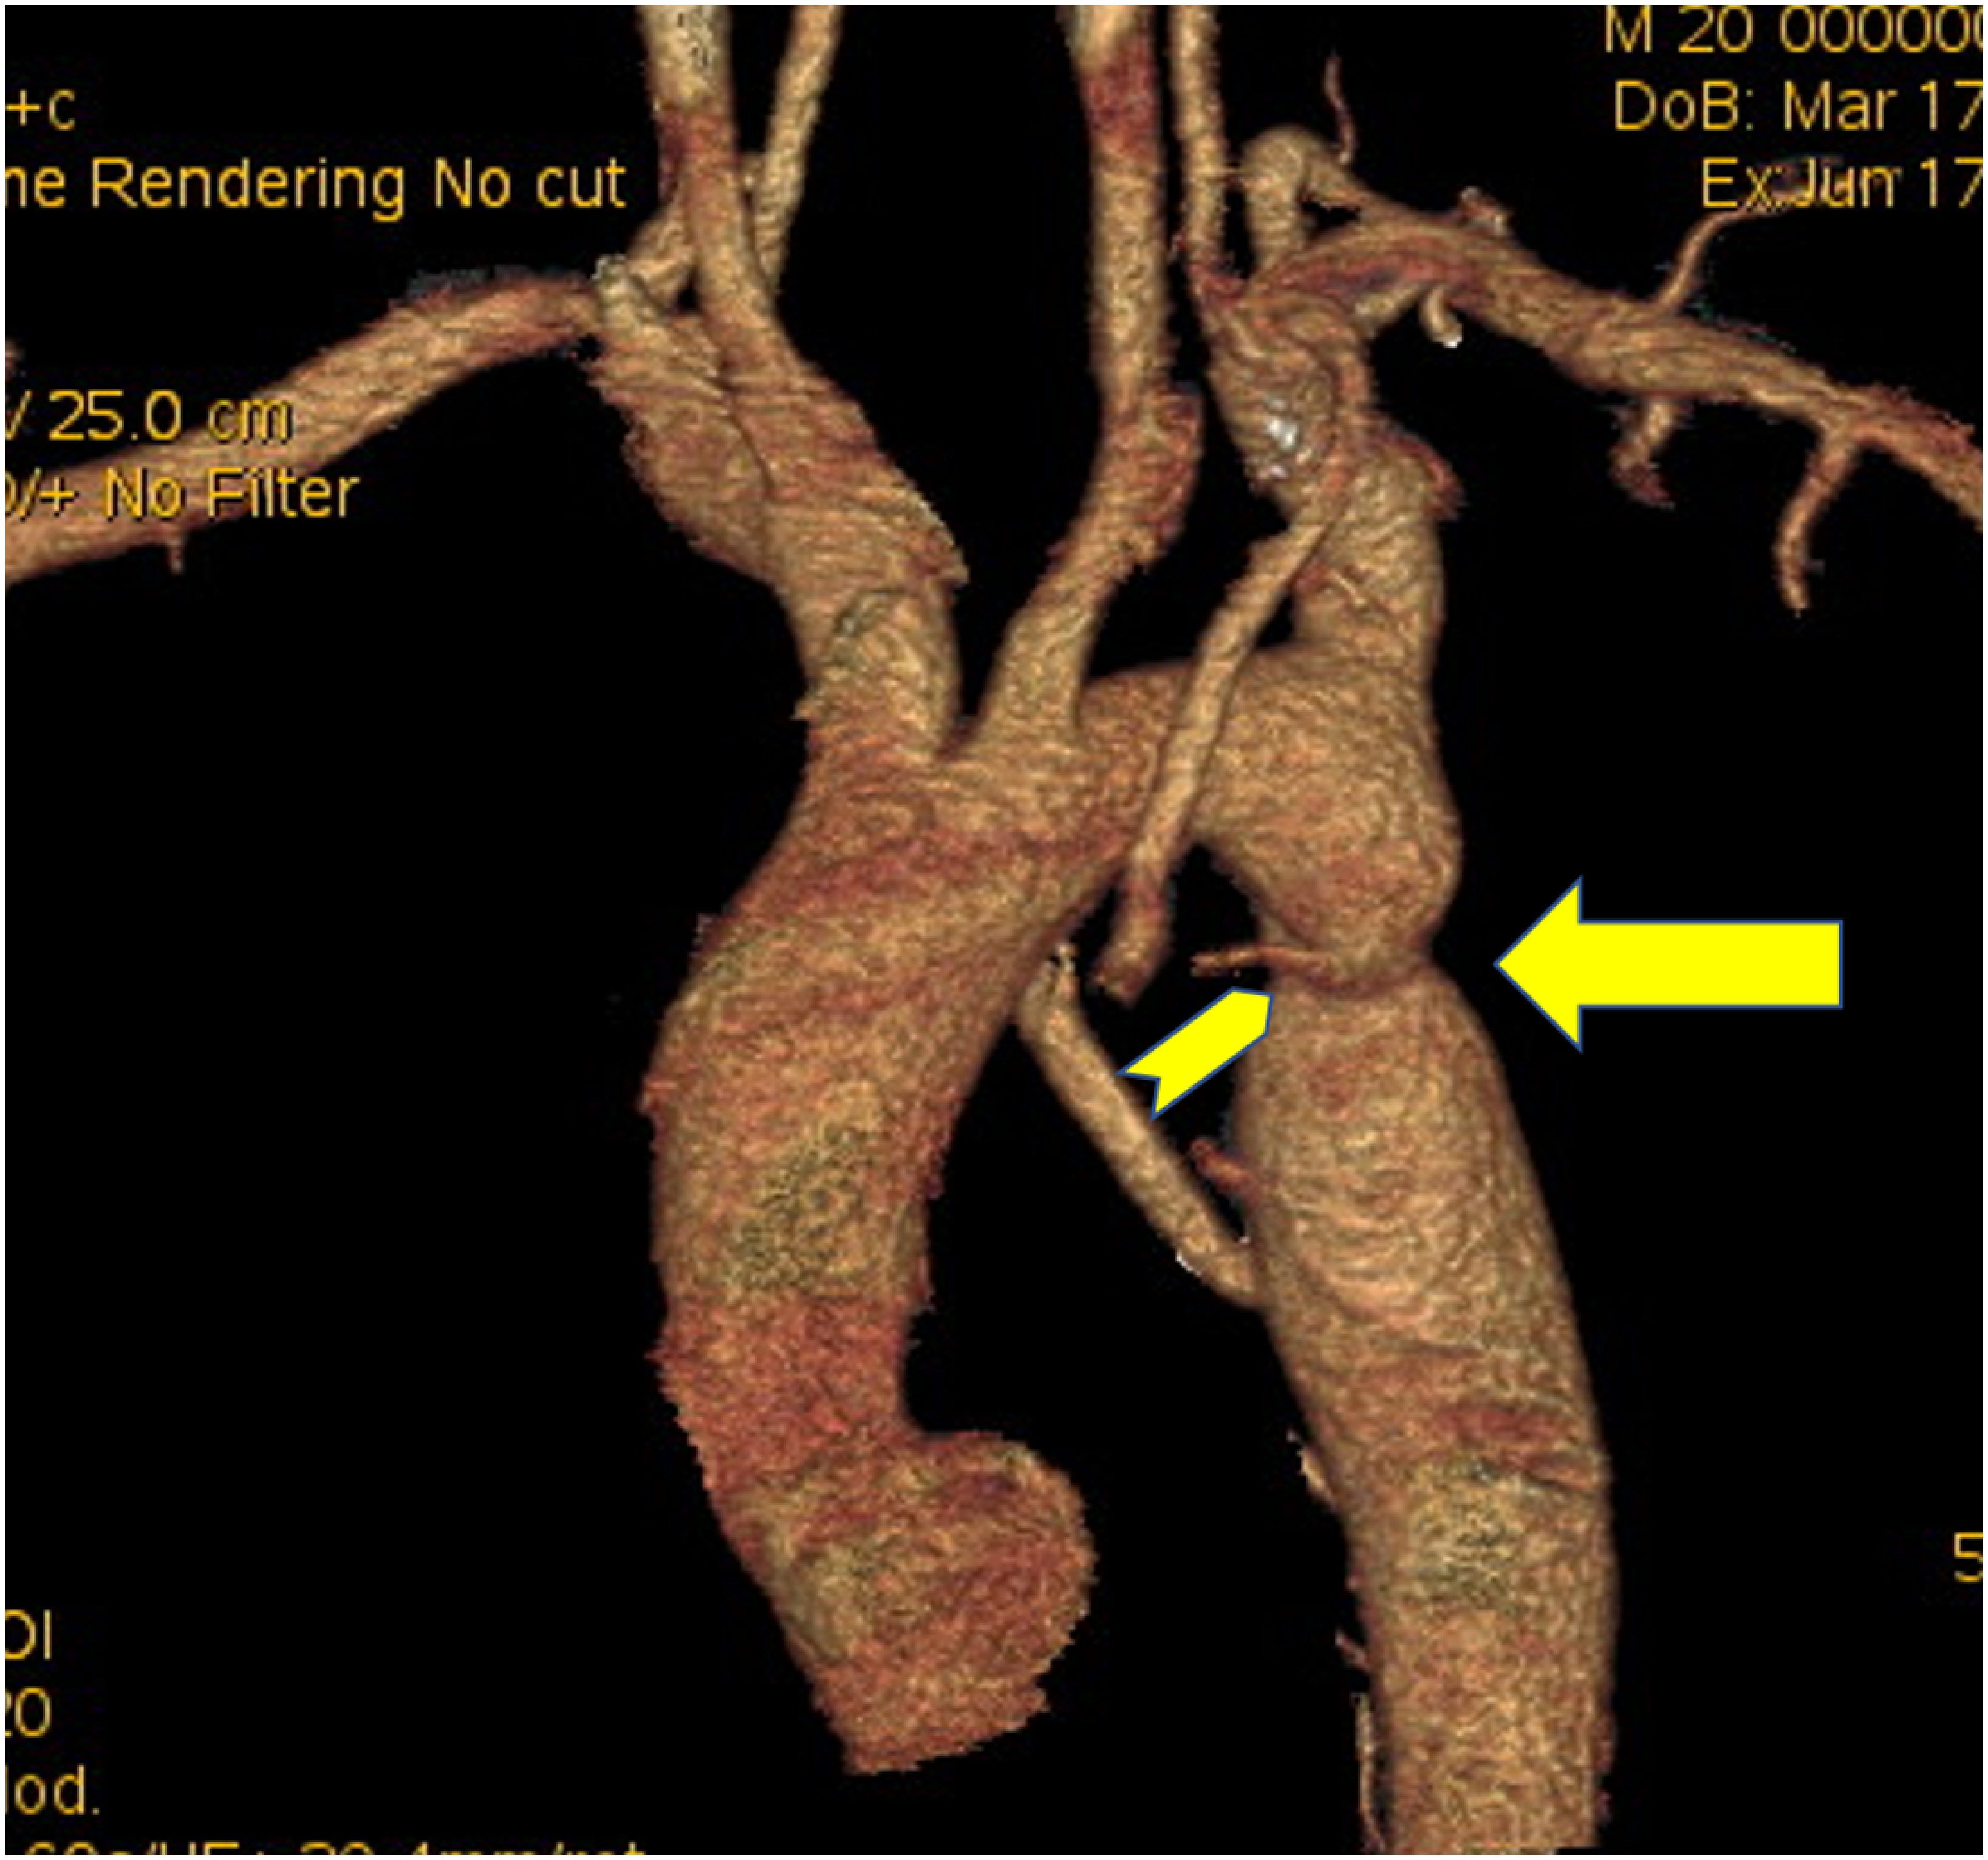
Fig. 1 Three-dimensional computed tomography of the thoracic aorta. The large arrow indicates coarctation of the aorta. The small arrow indicates patent ductus arteriosus located in the proximal portion of the coarctation.
